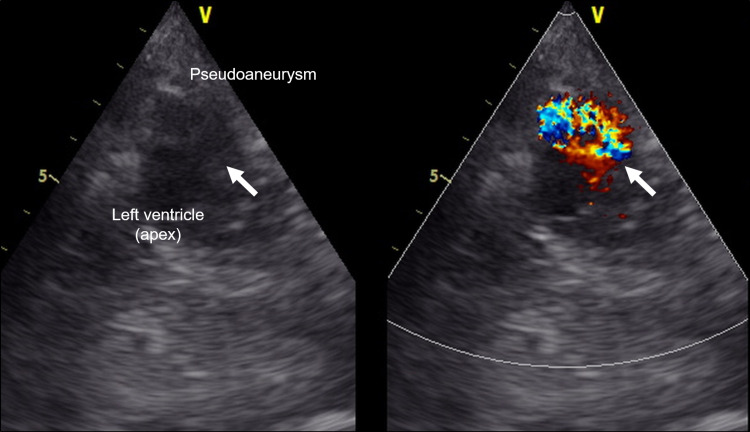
Transthoracic echocardiography taken at an emergency department A short-axis view of transthoracic echocardiography revealed a cavity at the apex. Color Doppler imaging showed abnormal blood flow from the left ventricle toward the cavity. The arrows indicate the orifice of the pseudoaneurysm.
